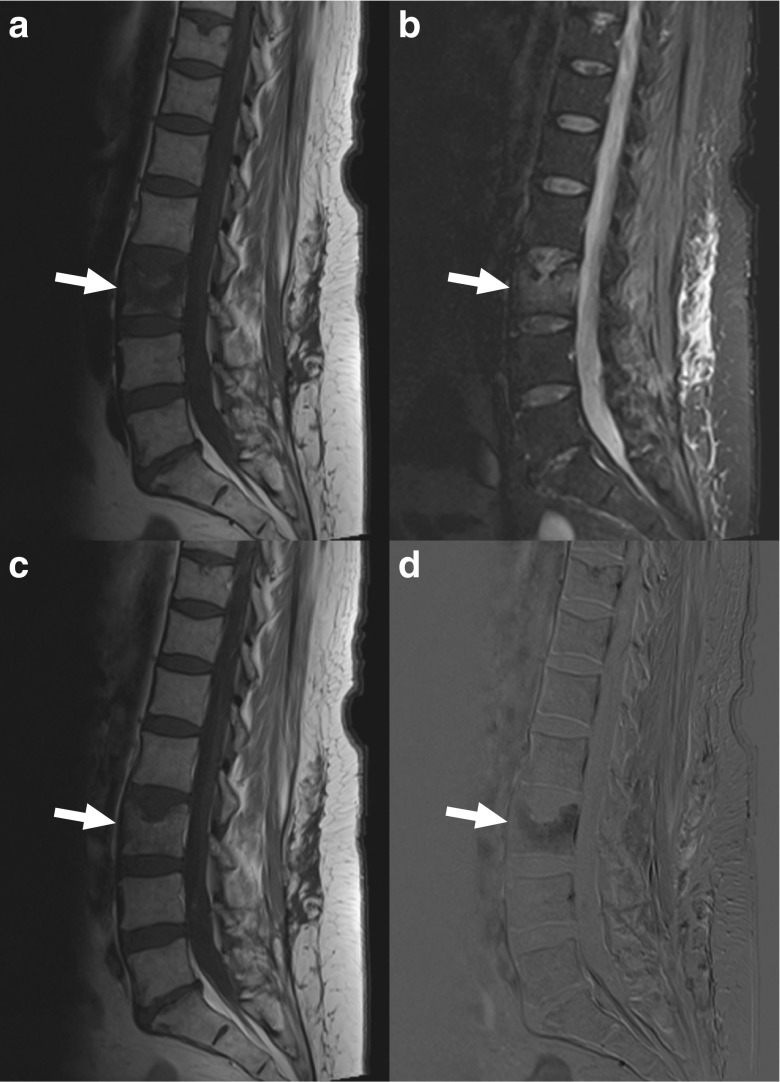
A 54-year-old woman with MRI findings consistent with acute Schmorl node. Sagittal T1-weighted (a), fat-suppressed T2-weighted (b), gadolinium-enhanced T1-weighted (c), and gadolinium-enhanced subtraction images (d) show a focal impression in the superior endplate of the L3 vertebra with surrounding pathological signal intensity (*arrows*), but involvement of only one endplate and no diffuse signal intensity abnormality of the adjacent disc. This patient underwent CT-guided biopsy with spondylodiscitis in the differential diagnosis of the original clinical report, but was excluded from this study
